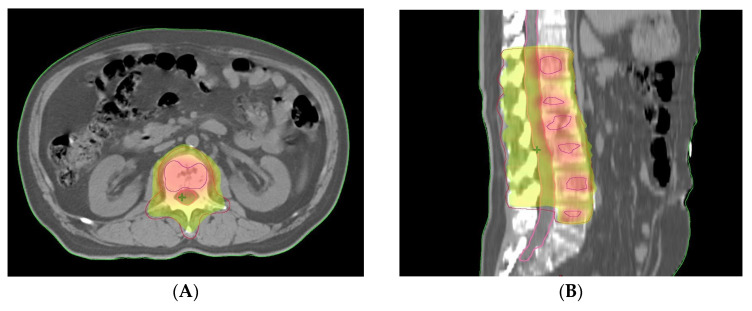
Intensity-modulated radiation therapy with the simultaneous integrated boost. Radiotherapy dose distribution in the (A) axial and (B) sagittal planes PET/CT scans are shown. Red area indicates the gross target volume: hypermetabolic lesions irradiated with a total dose of 40 Gy in 10 fractions. Yellow–green areas indicate the clinical target volume: residual vertebrae and spinous processes with a total dose of 30 Gy in 10 fractions.
